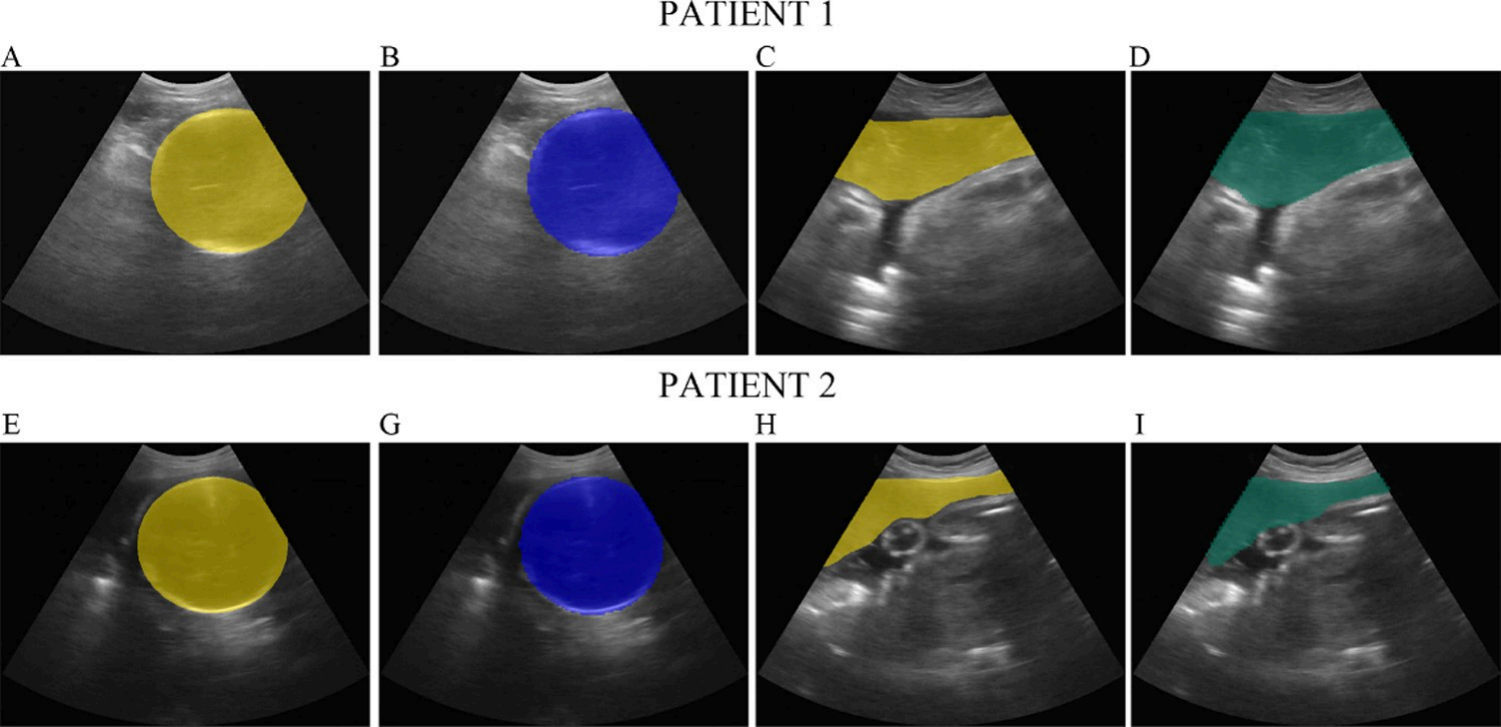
Ultrasound images. 2D ultrasound images from two representative patients (patient 1, upper row; patient 2, lower row) with overlaid color masks showing actual (yellow) versus predicted locations of the fetal head (blue) and placenta (green).
